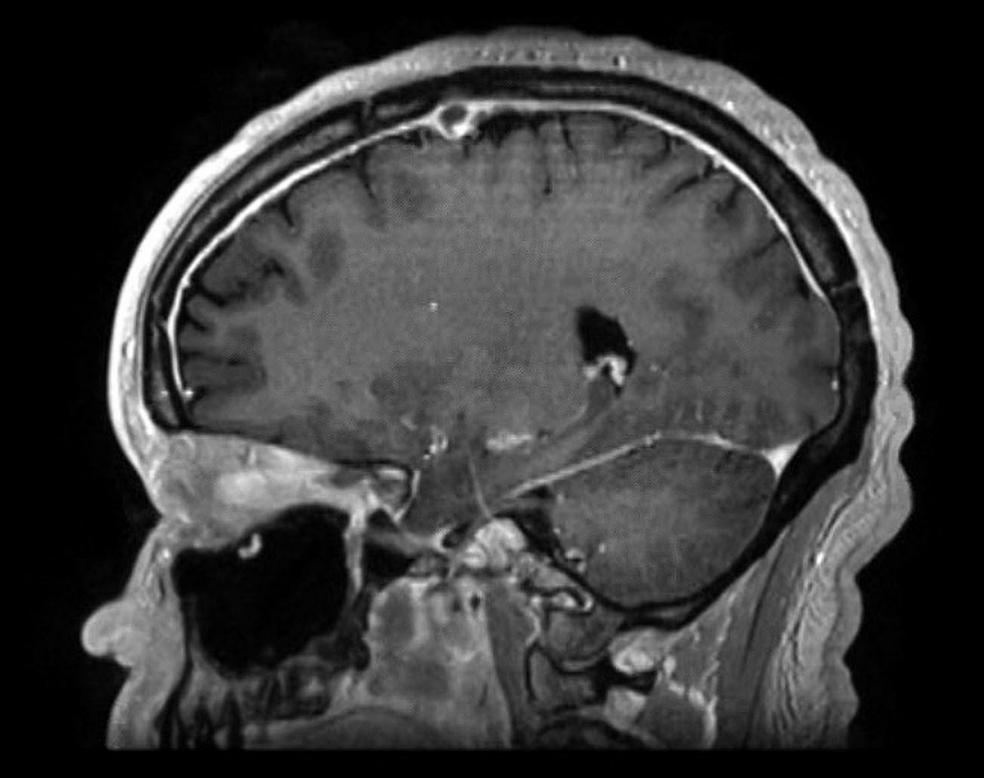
MRI brain: post-contrast sagittal sequence showing pachymeningeal enhancement.
